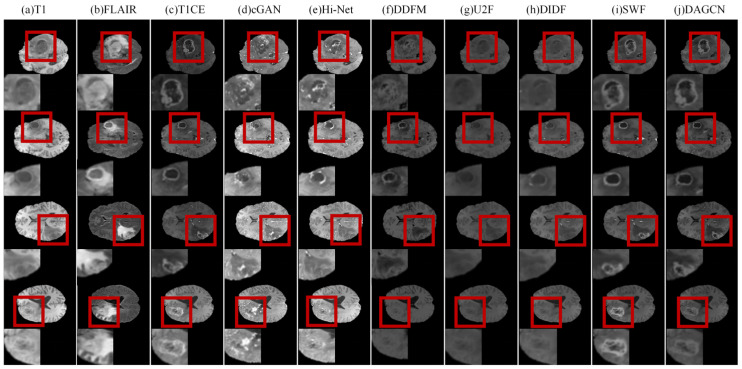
Visual comparison of synthesized T1-CE images under the T1 + FLAIR input setting. Results from cGAN, Hi-Net, DDPM, U2Fusion, DIDFuse, SwinFusion, and DAGCN are shown together with the real T1-CE reference. DAGCN produces cleaner background regions and more spatially focused lesion enhancement, particularly benefiting from the CSF-suppression property of FLAIR guidance.
